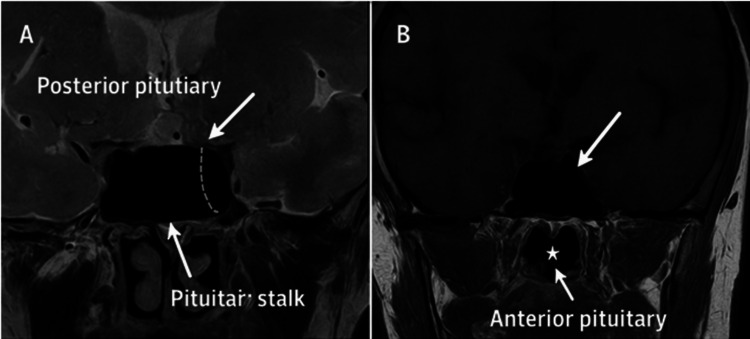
Normal pituitary MRI (A) Post-contrast T2-weighted coronal image shows posterior pituitary hyperintensity (arrow) (B) T1-weighted post-contrast coronal image demonstrates enhancing anterior pituitary (star) and stalk (arrowhead)
